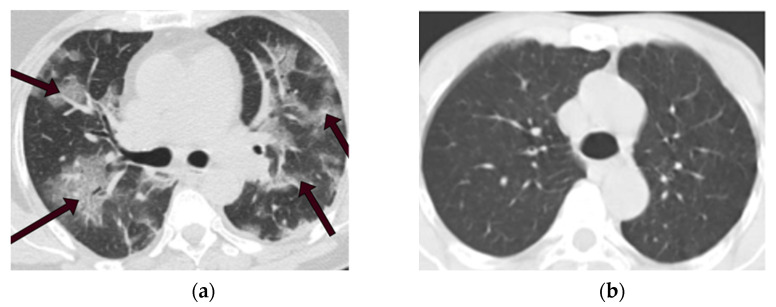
CT scans of COVID-19 and non-COVID-19 patients. (a) CT scan of a COVID-19 patient. (b) CT scan of a non-COVID-19 (not normal) patient.
